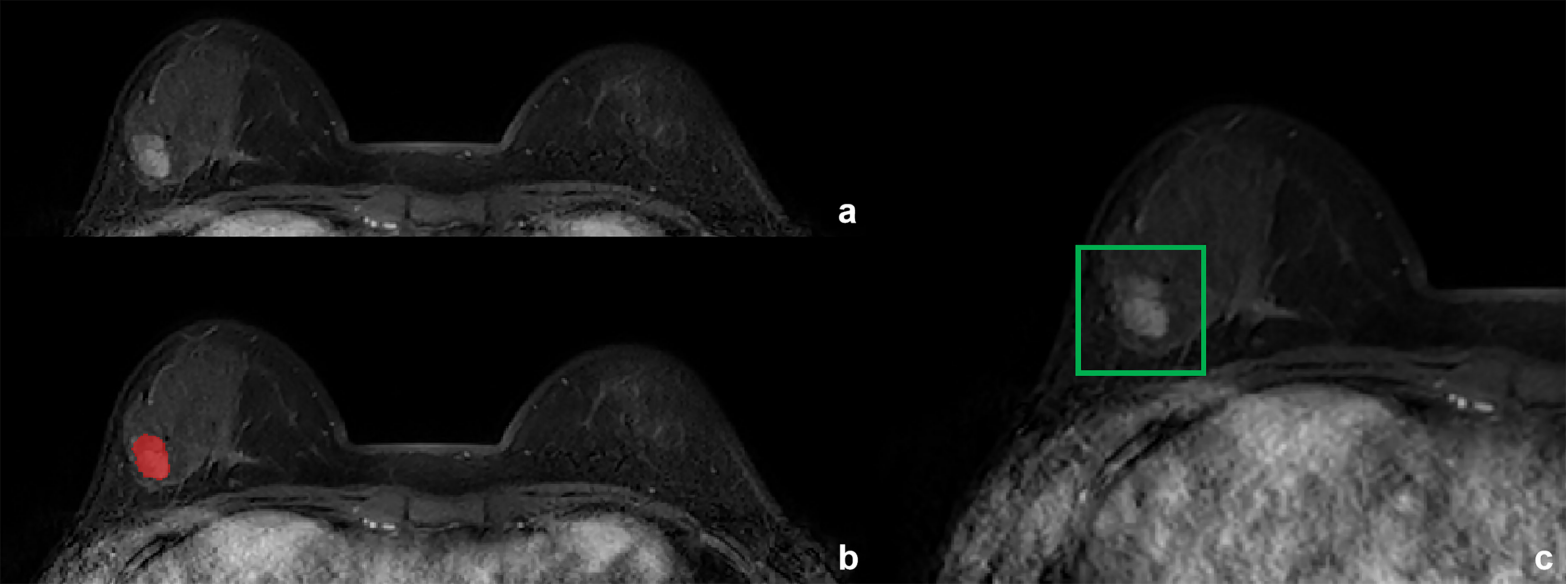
Fibroadenoma in a 29-year-old woman. (A) T1-weighted postcontract image. (B) Annotation masks (in red) are superimposed over the MR images. The annotations were made by radiologists using ITK-snap. (C) The anchor box was generated by the Faster R-CNN model after the segmentation.
